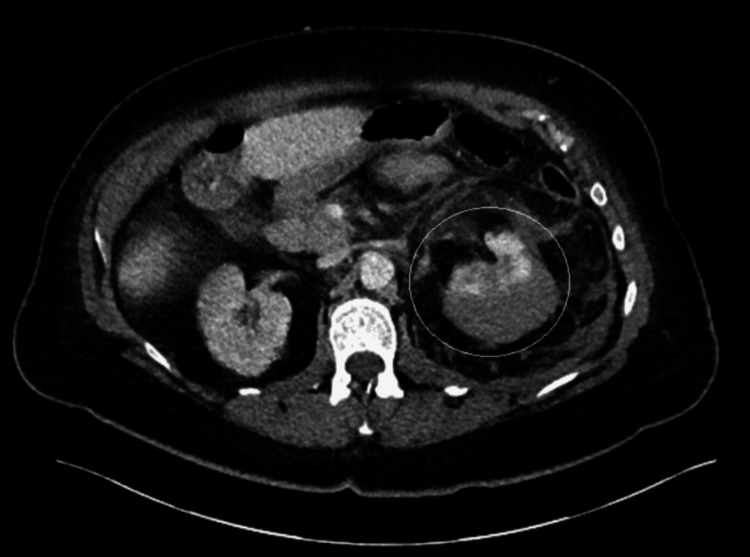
CT of the abdomen pelvis showing acute left perinephric/subcapsular hematoma CT: computed tomography
